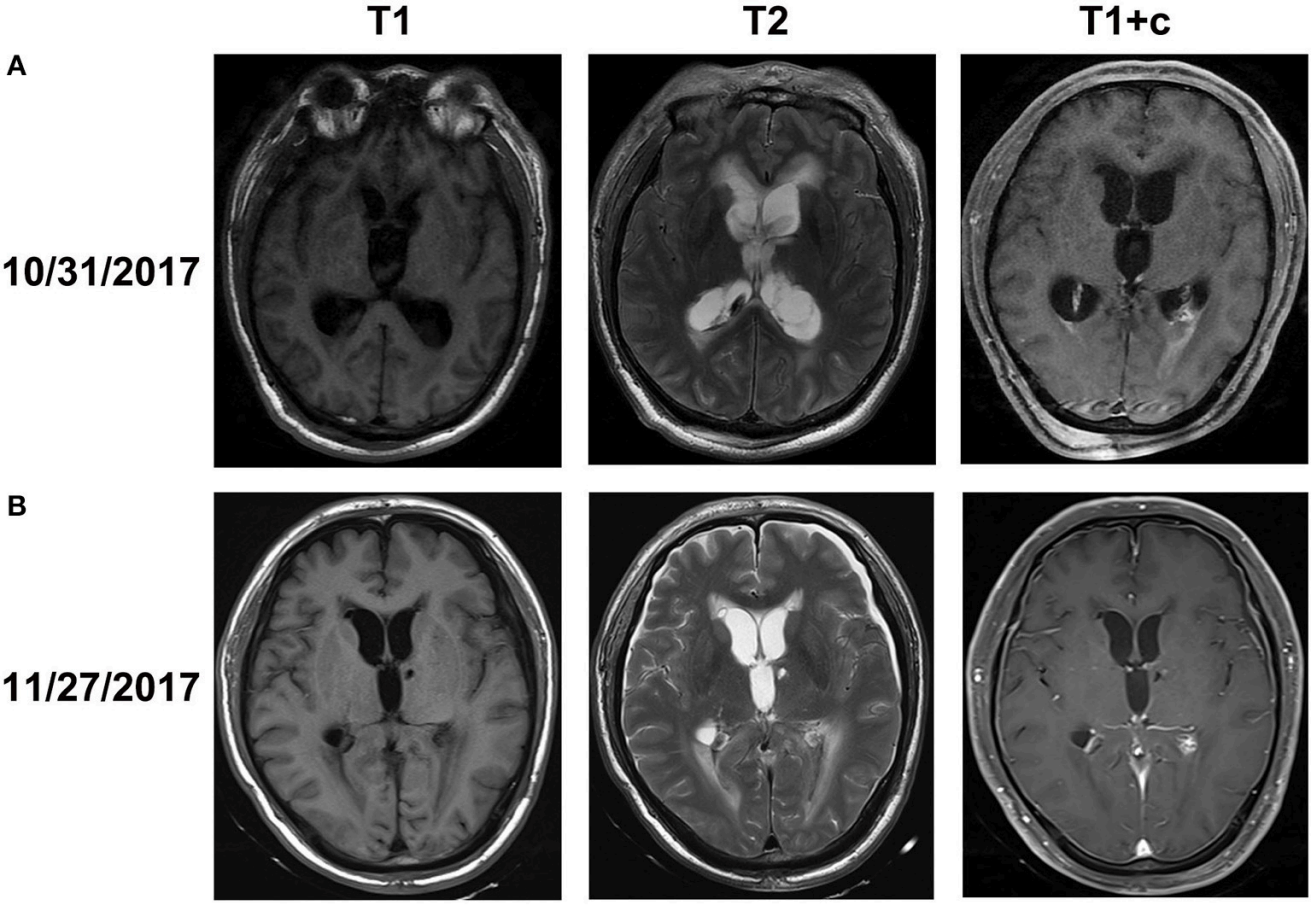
Brain MRI obtained before (A) and after (B) abscess clearance combined with tigecycline treatment (A). MRI on October 31, 2017 showed that interstitial cerebral edemas beside the ventricles and bilateral ventricular occipital horn enhancement along with hydrocephalus, which indicated signs of ventriculitis (B). MRI after abscess clearance combined with tigecycline treatment revealed the disappearance of the edemas, ventricular enhancement, and hydrocephalus.
